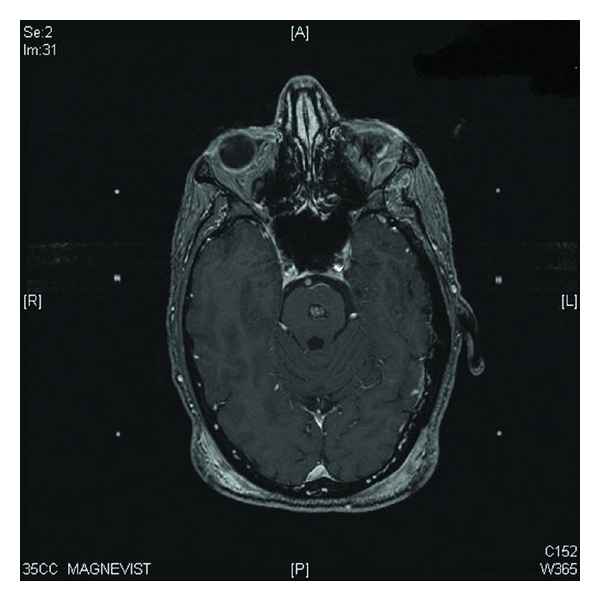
Axial T1 postgadolinium enhanced brain MRI with a visible pontine brain metastasis.
